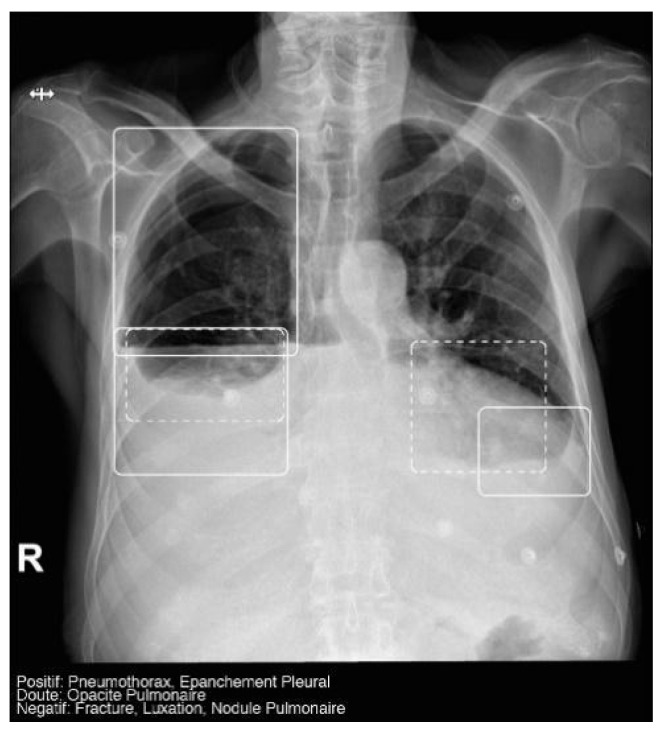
Chest radiograph with AI analysis, which detected a positive right pneumothorax, positive bilateral pleural effusion (both outlined with continuous boxes), and doubtful bibasilar opacities (outlined with discontinuous boxes).
